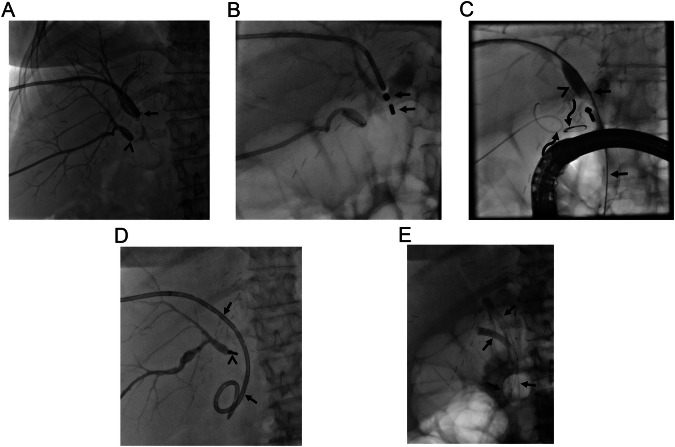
A 59-year-old woman underwent living donor liver transplantation surgery 10 months ago, and presented with pruritus and cholangitis. Liver MRI (not shown) demonstrated biliary obstruction. A Initially performed percutaneous biliary drainage revealed obstruction of two duct-to-duct anastomoses at the recipient’s main bile duct (arrow) and cystic duct (arrowhead). Both antegrade and retrograde attempts failed in traversing the obstruction. B First, magnets are placed at the anastomosis between the transplanted liver and the recipient’s main bile duct (arrows). C–E On the 5th day MCA was formed and antegrade wire access through the main bile duct was achieved (arrows, C). A balloon catheter (arrowhead, C) was used to push down the magnets. Consequently, a biliary drainage catheter was able to be placed (arrows, D). The second procedure was planned for MCA for cystic duct anastomosis obstruction (curved arrows, C). On the 6th day following magnet placement at cystic duct anastomosis (arrowhead, D), two fully covered self-expandable metallic biliary stents were able to be placed (arrows, E) and percutaneous drains were removed
